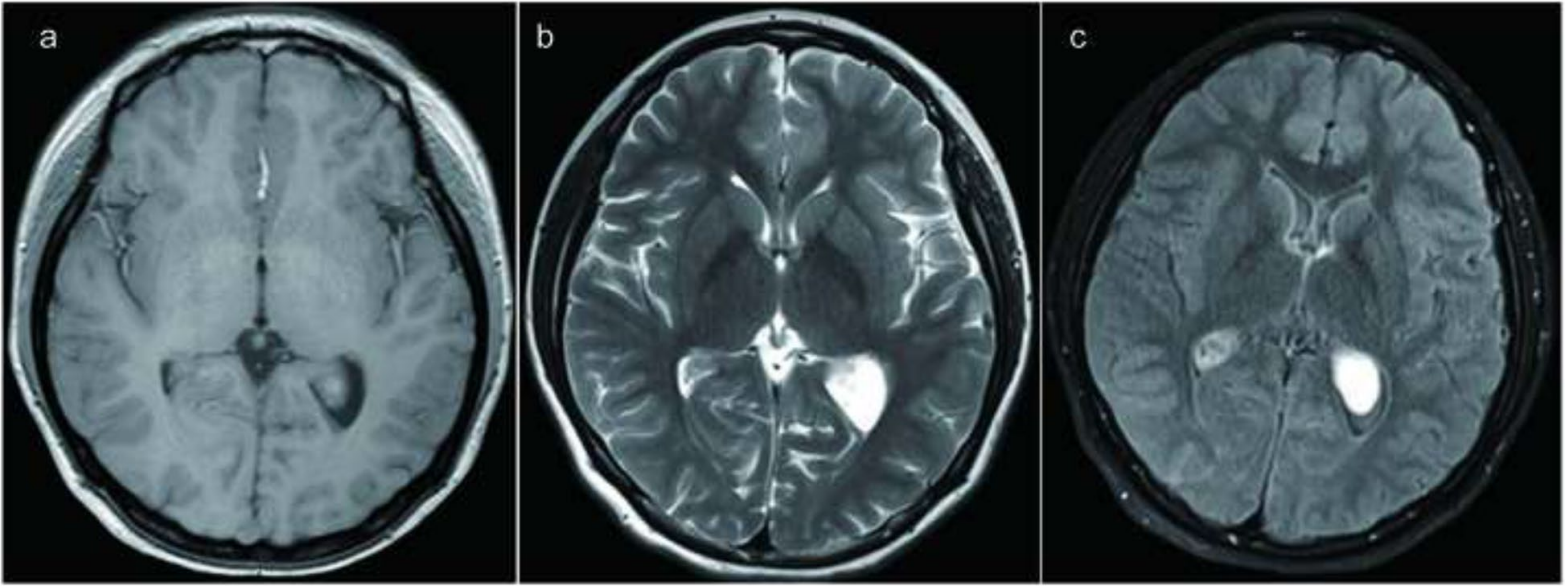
Initial magnetic resonance imaging. a Low-intensity area was evident in the bilateral lateral ventricles on T1-weighted images. b Low intensity area was seen on T2 weighted images. c Low intensity area was seen on fluid attenuated inversion recovery images
